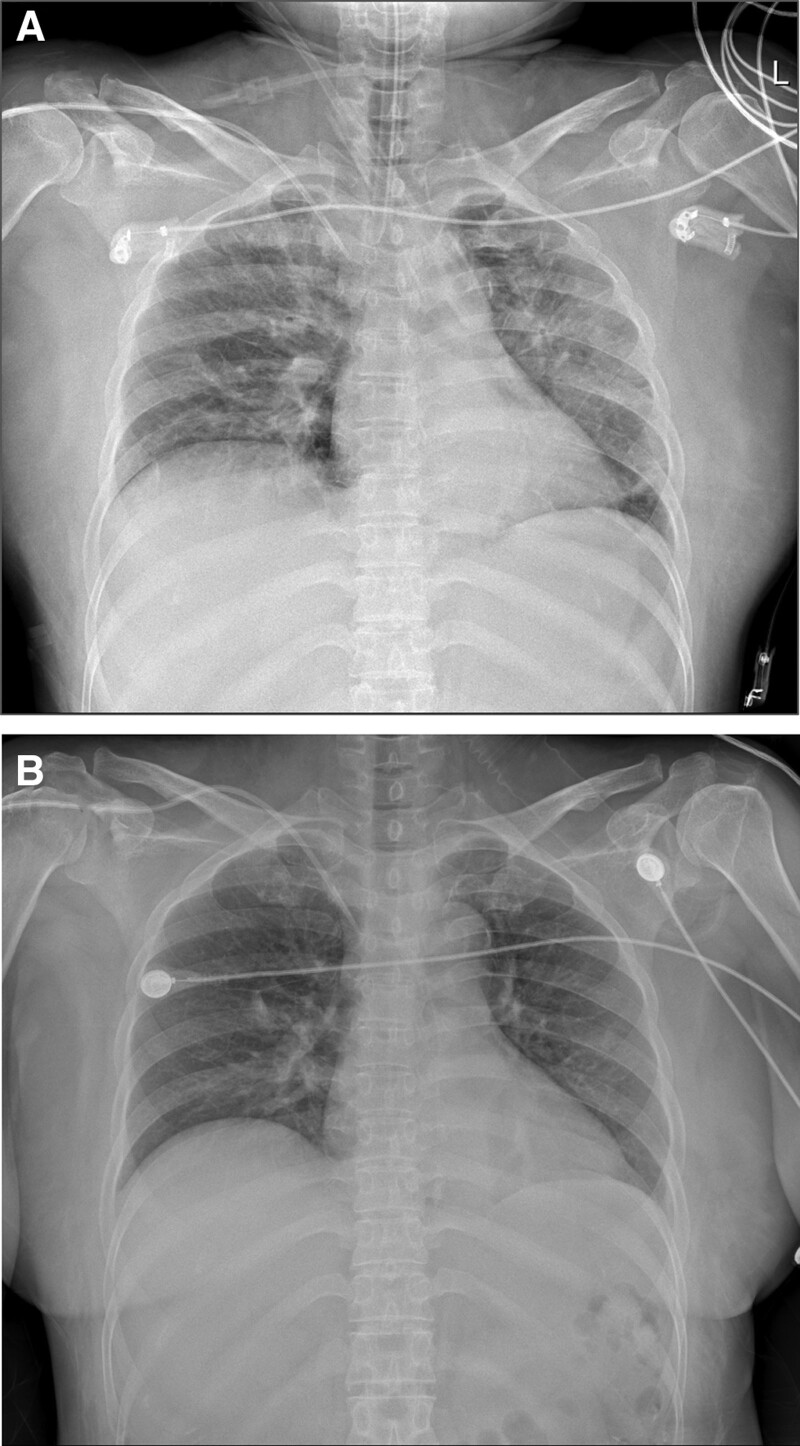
Comparison of anteroposterior chest radiographs immediately after transfer to the surgical intensive care unit (A) and 10 h post-surgery (B).
